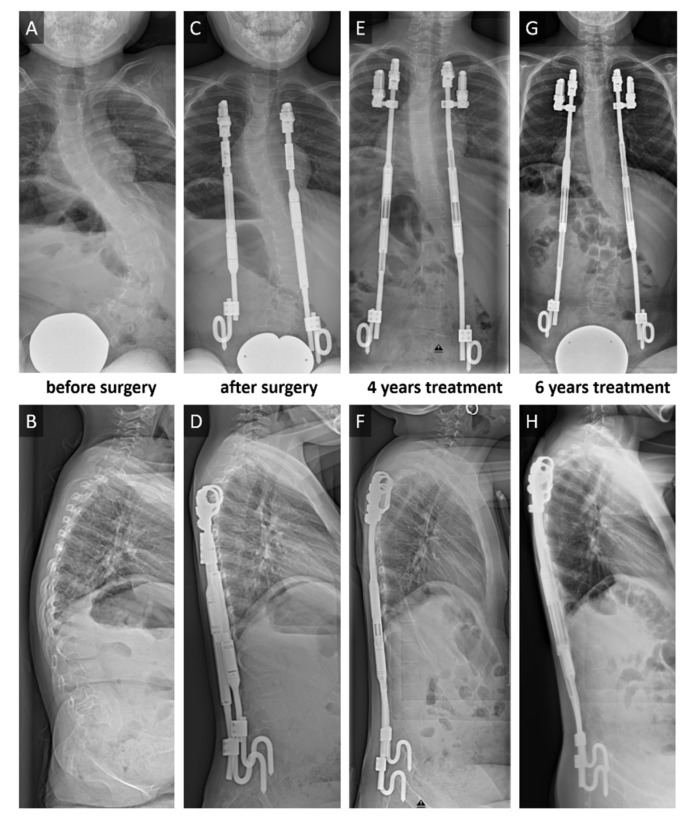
Bilateral rib-to-pelvis fixation with magnetically controlled growing rods (MCGR) in a 5-year-old spinal muscular atrophy type II girl with progressive scoliosis. Posterior anterior (p.a.) (A) and lateral (B) sitting radiographs before the surgical intervention. Deformity correction (C/D) after surgical treatment. Implant exchange after maximal distraction after 3 years of treatment. 4-year follow-up (E/F) with second MCGRs. 6-year follow-up (G/H) with fully distracted rods.
